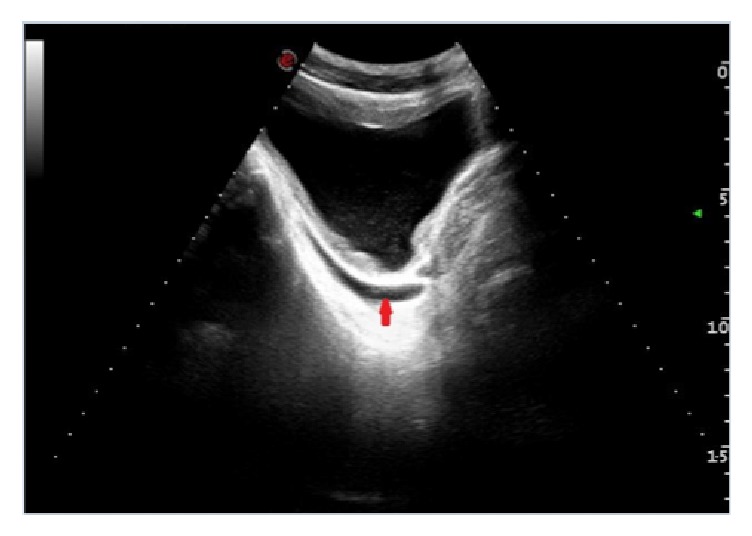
Abdominal ultrasound.* Bladder wall thickening (red arrow).
